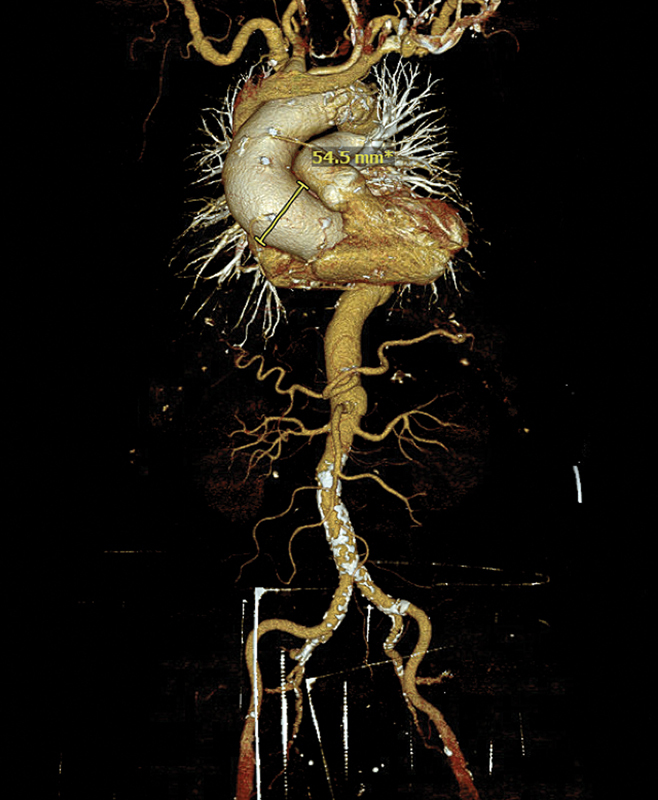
Computed tomographic angiogram of a patient with temporal arteritis and thoracic aortic aneurysm. Three-dimensional reconstruction demonstrates a large ascending aortic aneurysm measuring 54.5 mm in a patient who underwent surgical repair.
